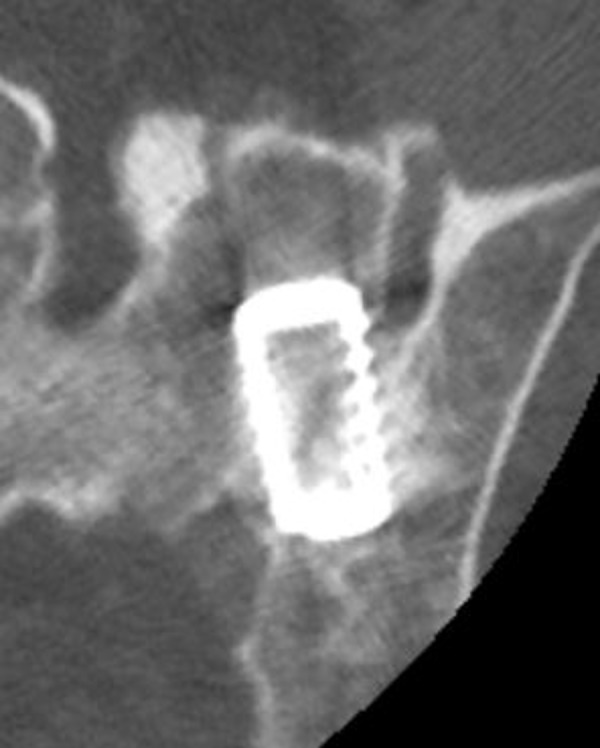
Axial CT of the left-sided posterior lateral oblique fusion
